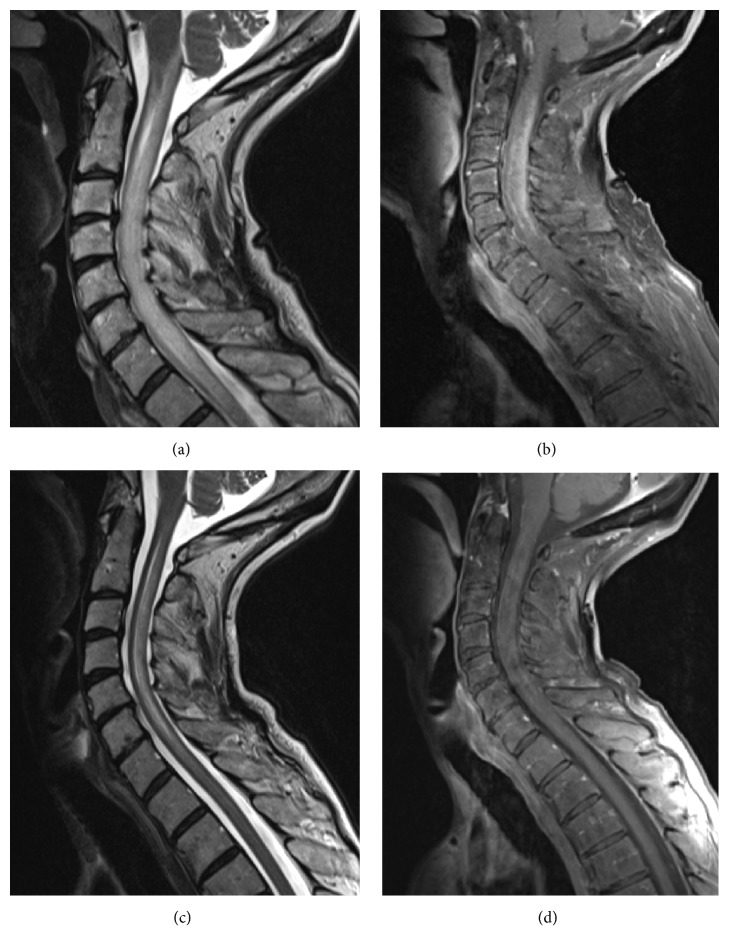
(a) Distinct oedema of the cervical spinal cord on T2-weighted sequences with spinal cord swelling and expansion into the brainstem. (b) Distinct gadolinium enhancement of the cervical spinal cord on T1-weighted sequences with cord swelling. (c) Regressive edema in the cervical spinal cord after 5 days of methylprednisolone followed by plasmapheresis and immunoadsorption. (d) Regressive gadolinium enhancement in the cervical spinal cord after therapy.
